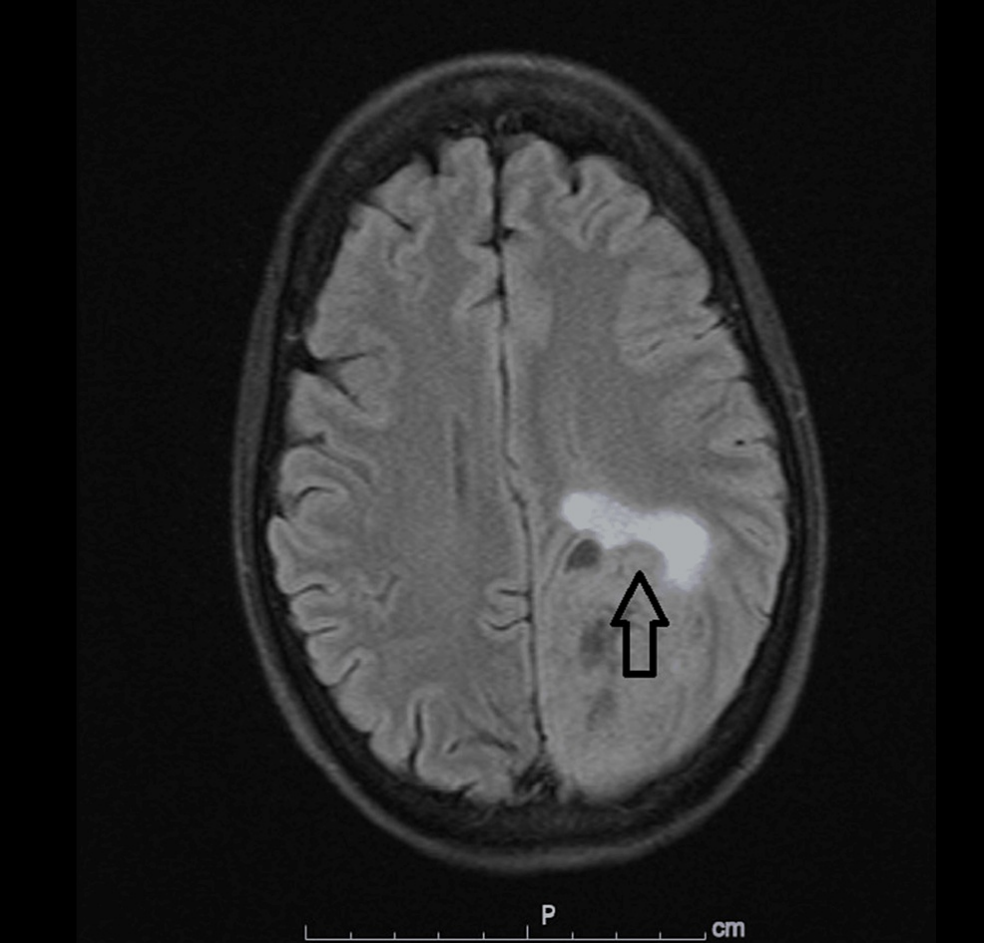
MRI Brain Magnetic resonance imaging (MRI) brain also showed evidence of a 6.4 cm left parietal-occipital mass (arrow).
